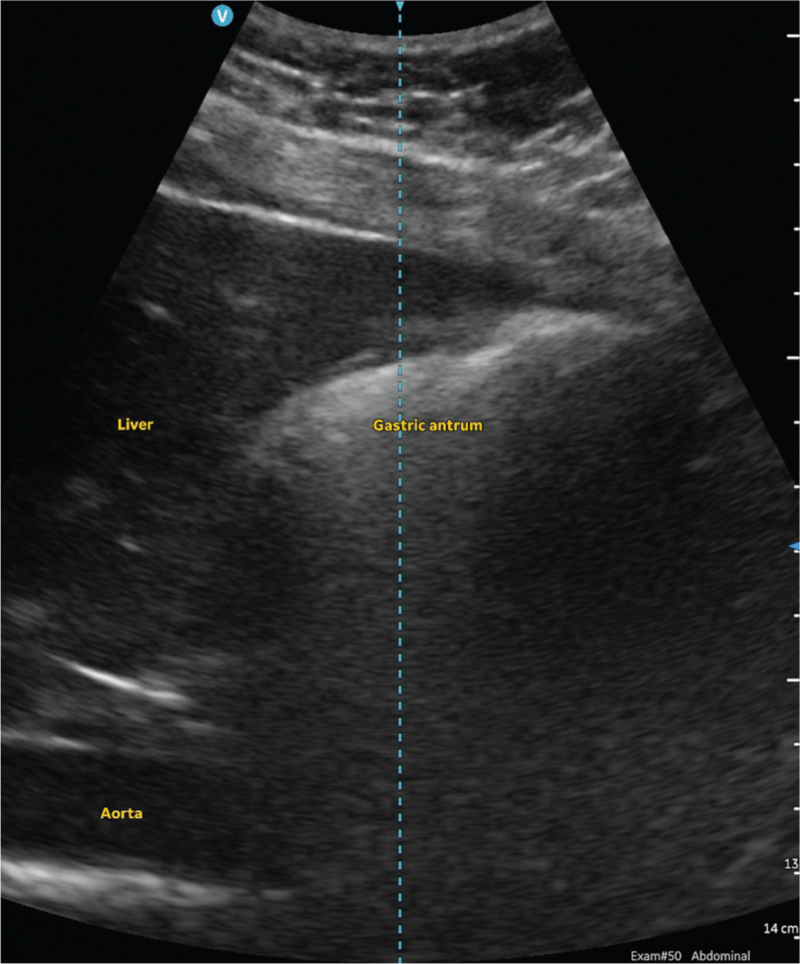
Ultrasound image of the gastric antrum following the ingestion of solids in a patient in the first trimester of pregnancy.
